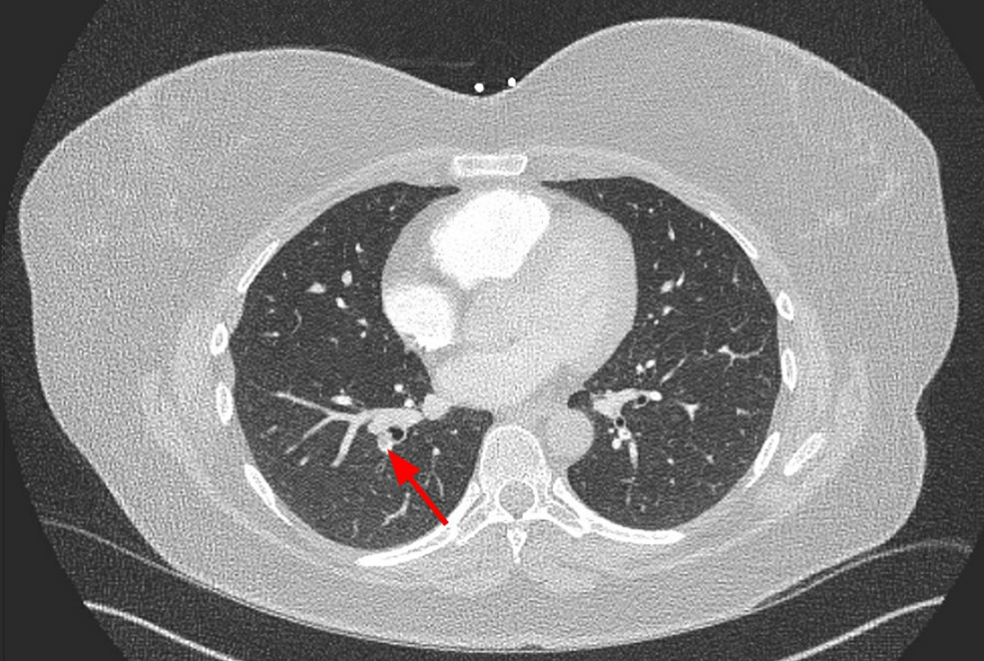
CTA showing thrombus in the segmental branch (red arrow) of the right inter-lobar artery. CTA, computed tomography angiography
